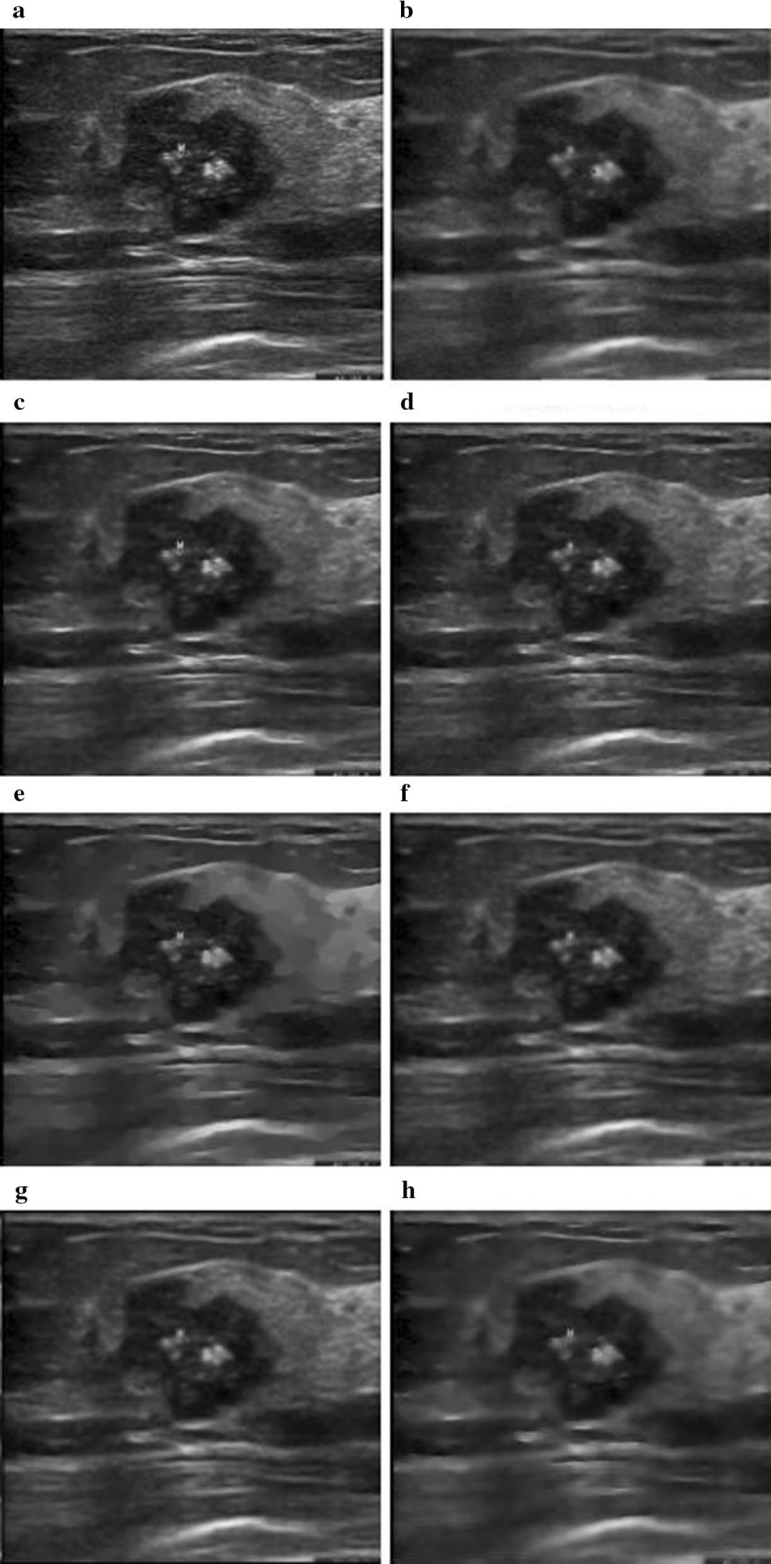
The visual experiment results for a the clinic breast ultrasound image, the output by b the proposed RSBF, c SBF filter, d Median filter, e SRAD, f NL-means, g Lee filter and h Kuan filter
